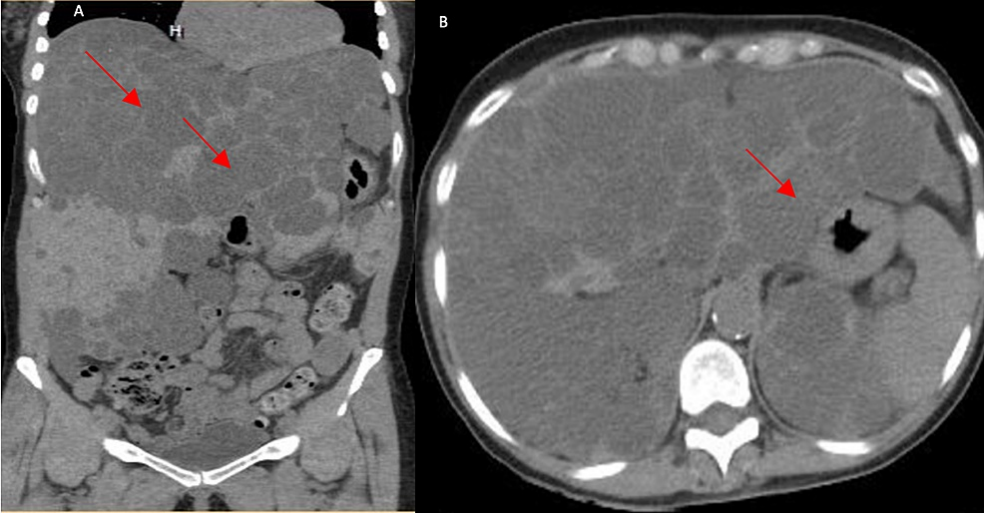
Non-contrast computed tomography coronal view (A) and axial view (B) of the abdomen showing hyperdense cysts within the parenchyma of the liver and a diffusely enlarged liver (red arrow).
